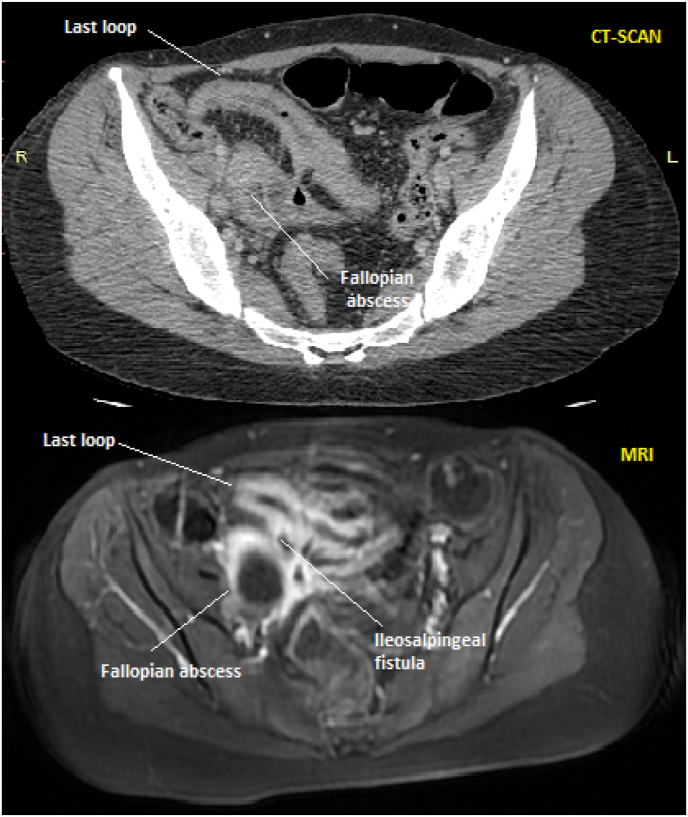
Abdominal CT-scan* showing an abscess of the right fallopian tube that was adjoining the last loop. *Abdominal MRI* revealing an abnormal connection between the right salpinx and the last loop with T2 hypersignal.
